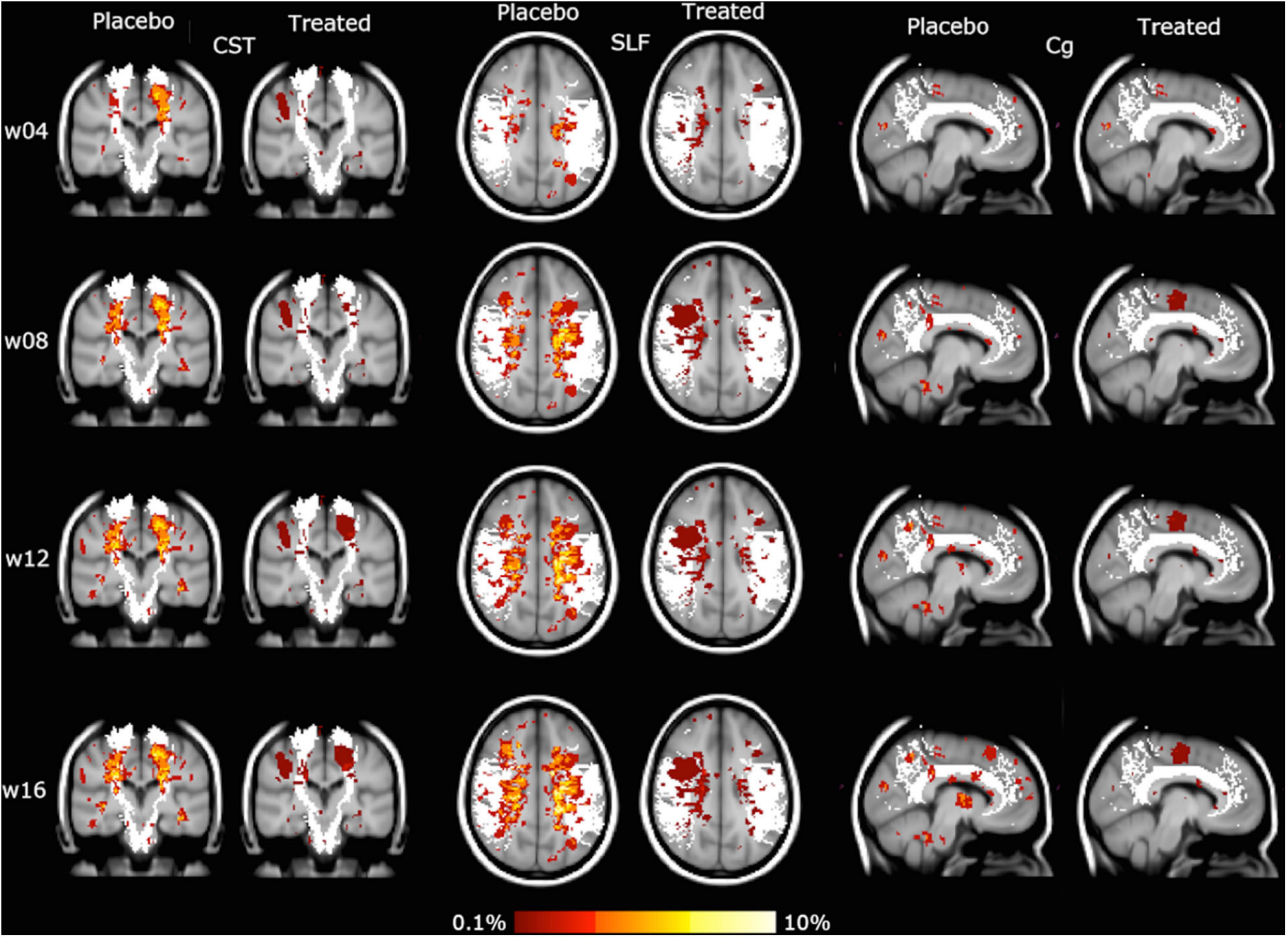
Lesion probability map (LPM) of the spatial distribution of active MRI lesions, expressed as combined unique active (CUA) lesions, mapped on some representative white matter (WM) tracts (in white: corticospinal tract [CST], superior longitudinal fascicle [SLF], cingulum [Cg]). Red-to-yellow represents the frequency of CUA lesion occurrence (range: 0.1–10%). The background image is the study template in standard space (MNI 2 mm^3^).
